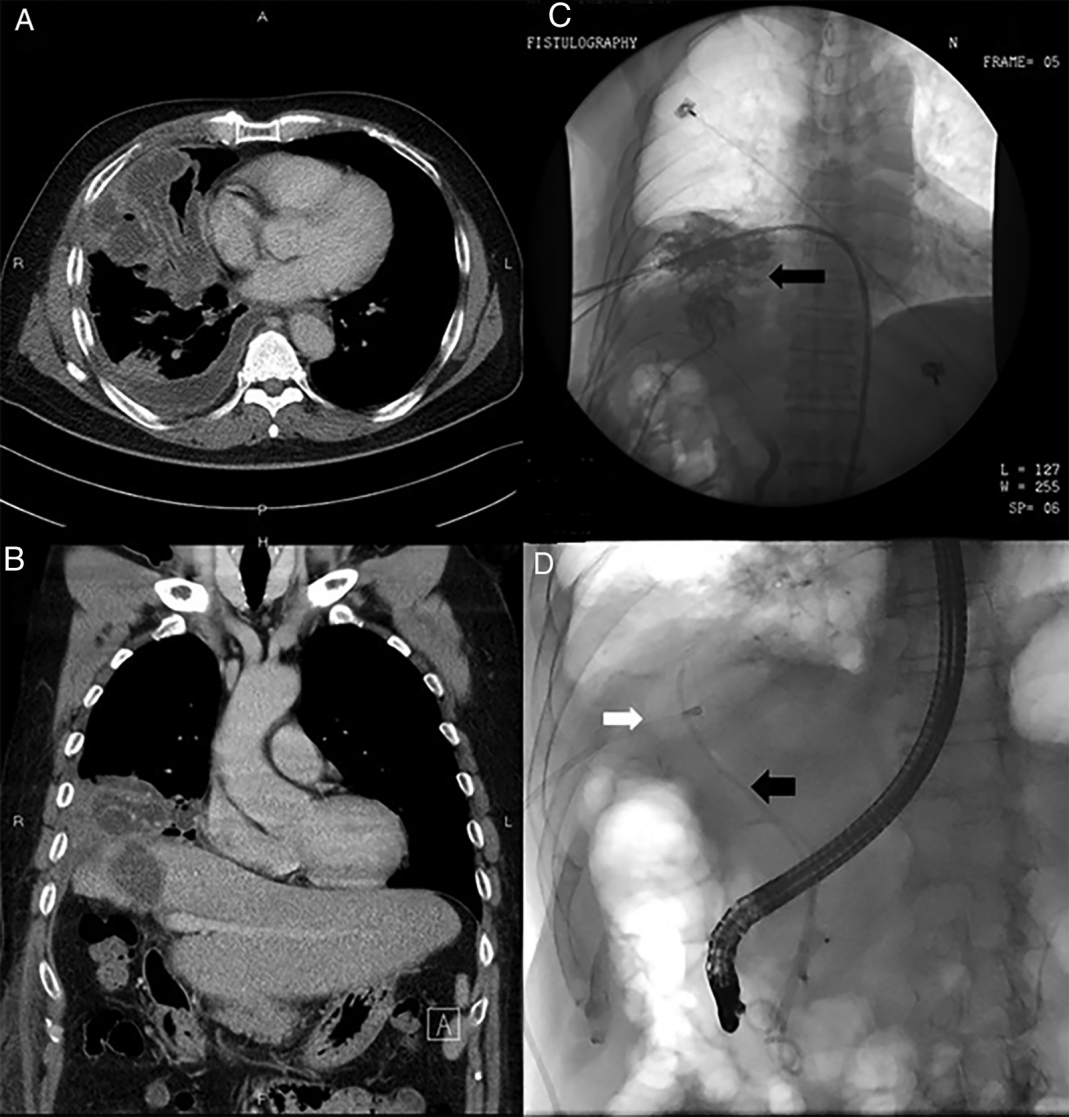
(A) Computed tomography demonstrated right middle lobe consolidation with pleural effusion. (B) Right subphrenic fluid collection. (C) Percutaneous cholangiography indicated the bronchobiliary fistula. (D) Percutaneous drainage (white arrow) combined with endoscopic retrograde biliary drainage (black arrow).
